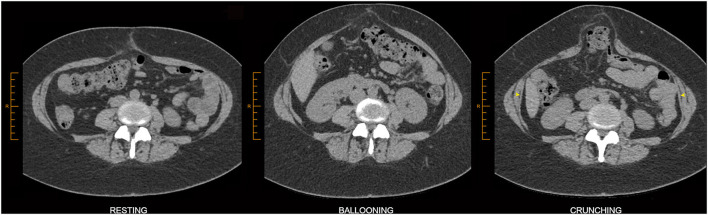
Value of crunching CT acquisition. This example of midline ventral hernia is best demonstrated on the crunching image. Crunching images are *either* equivalent *or* superior to ballooning images for the demonstration of ventral hernias in a great majority of cases. Associated TA muscle dysfunction can be appreciated here as relatively thin TA muscles on the resting image that show relatively poor contractility on the crunching image (yellow arrowheads). The straining images in this case also appear to show stretch of the linea semilunaris, a finding that has not yet been investigated for reliability but which might nevertheless raise *suspicion* of abnormal fascial compliance.
